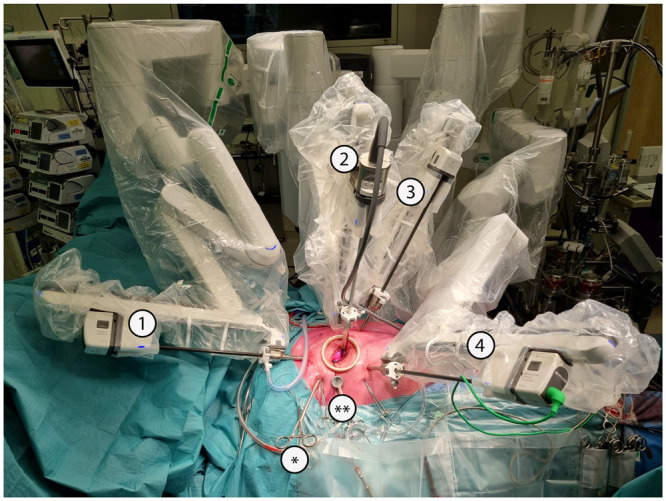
Robotic system (Intuitive Da Vinci X) docked to the patient. It is important to connect all 4 arms in the direction they will move, to avoid conflict with and between other structures, both inside and outside the chest. The camera port is connected to arm number 2 (but can also be connected to any of the other arms in the newer systems) and, under endoscopic or direct visualization, surgical instruments operated by the right and left hands of the console surgeon are typically placed in arms number 1 and 4 and introduced by the patient side assistant for control by the console surgeon, depending on the system used. The atrial retractor is inserted under endoscopic vision on the remaining arm (arm number 3). * The transthoracic aortic clamp is inserted through a separate stab wound incision.  A suction catheter to vent the left heart chambers can be inserted through the working port or via a separate port.
